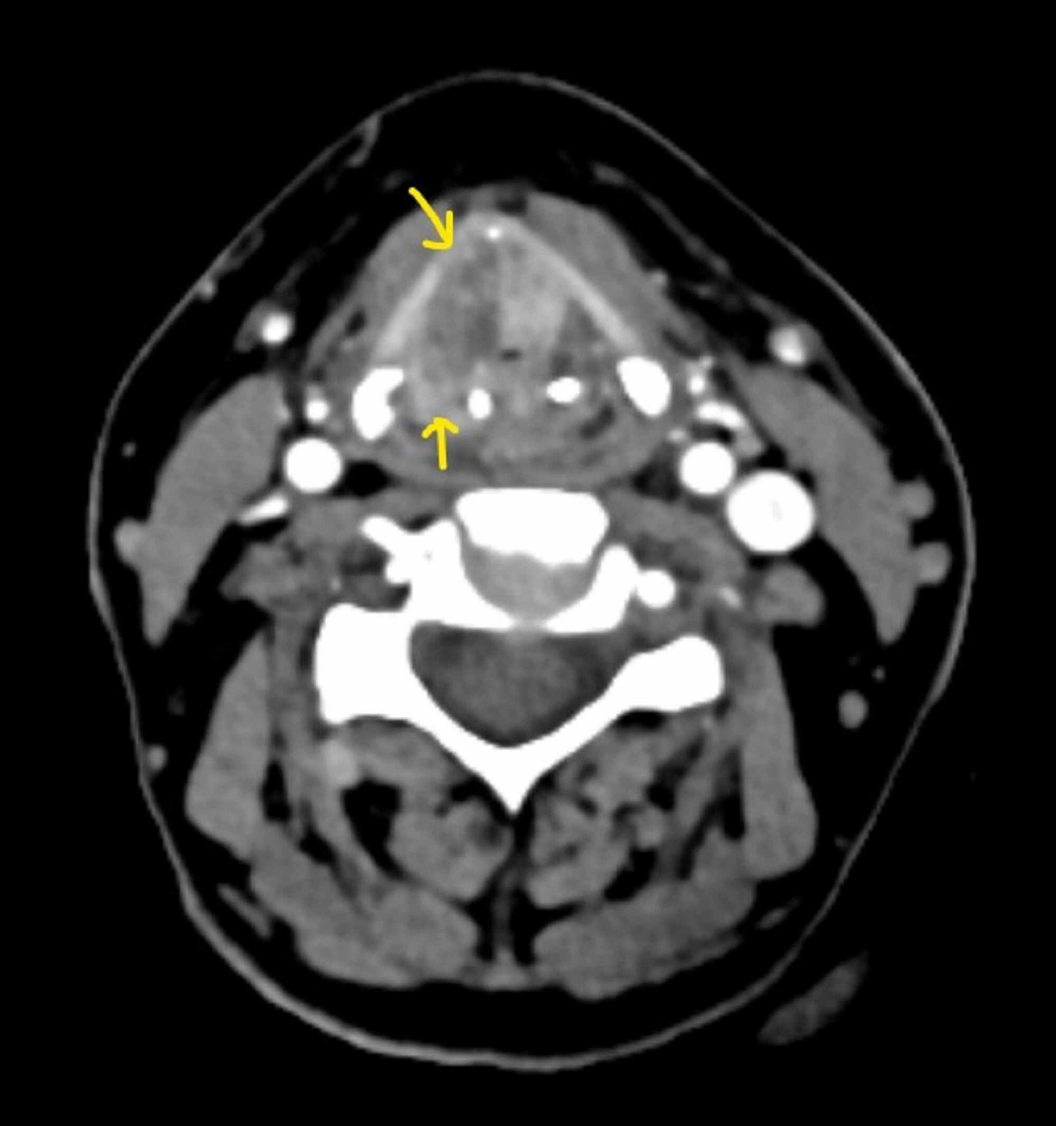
Radiological image (axial cuts) Axial cut, soft tissue window contrast computed tomography of the neck showing a heterogeneously enhancing lesion of both sides of the supraglottis extending to the right pyriform sinus (lower arrow) invading the thyroid cartilage (upper arrow).
